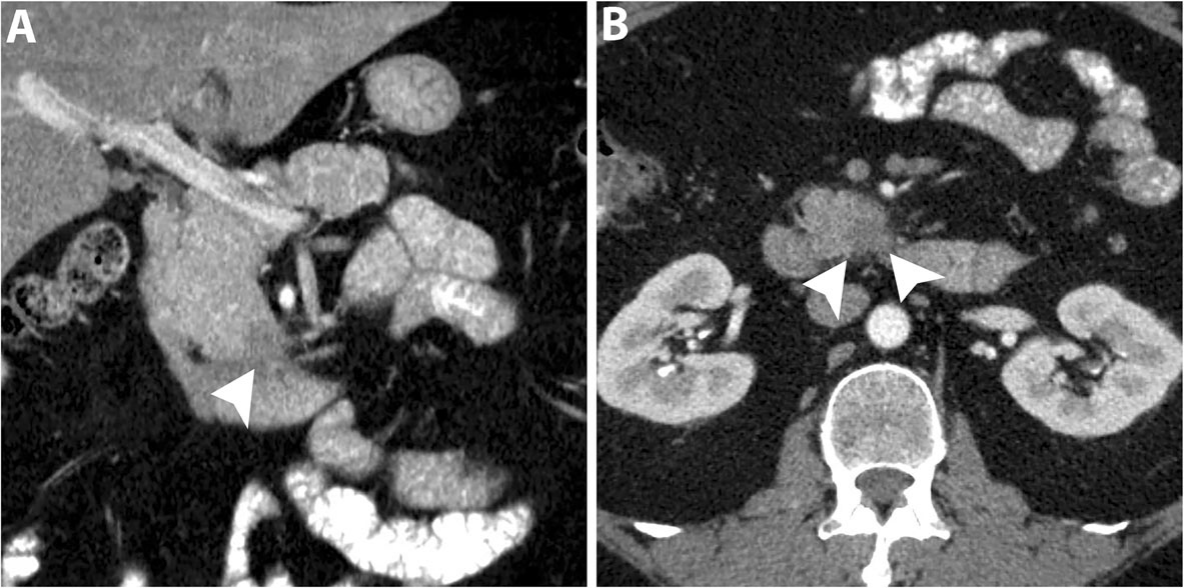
A 47-year-old man with uncinate process PDAC. Axial (a) and coronal (b) pancreatic phase CT images show a small hypoattenuating mass (arrowheads) in the uncinate process of the pancreas. Due to the location of the tumor, there is no CBD or MPD dilatation. EUS-guided biopsy revealed PDAC and the patient underwent pancreaticoduodenectomy
